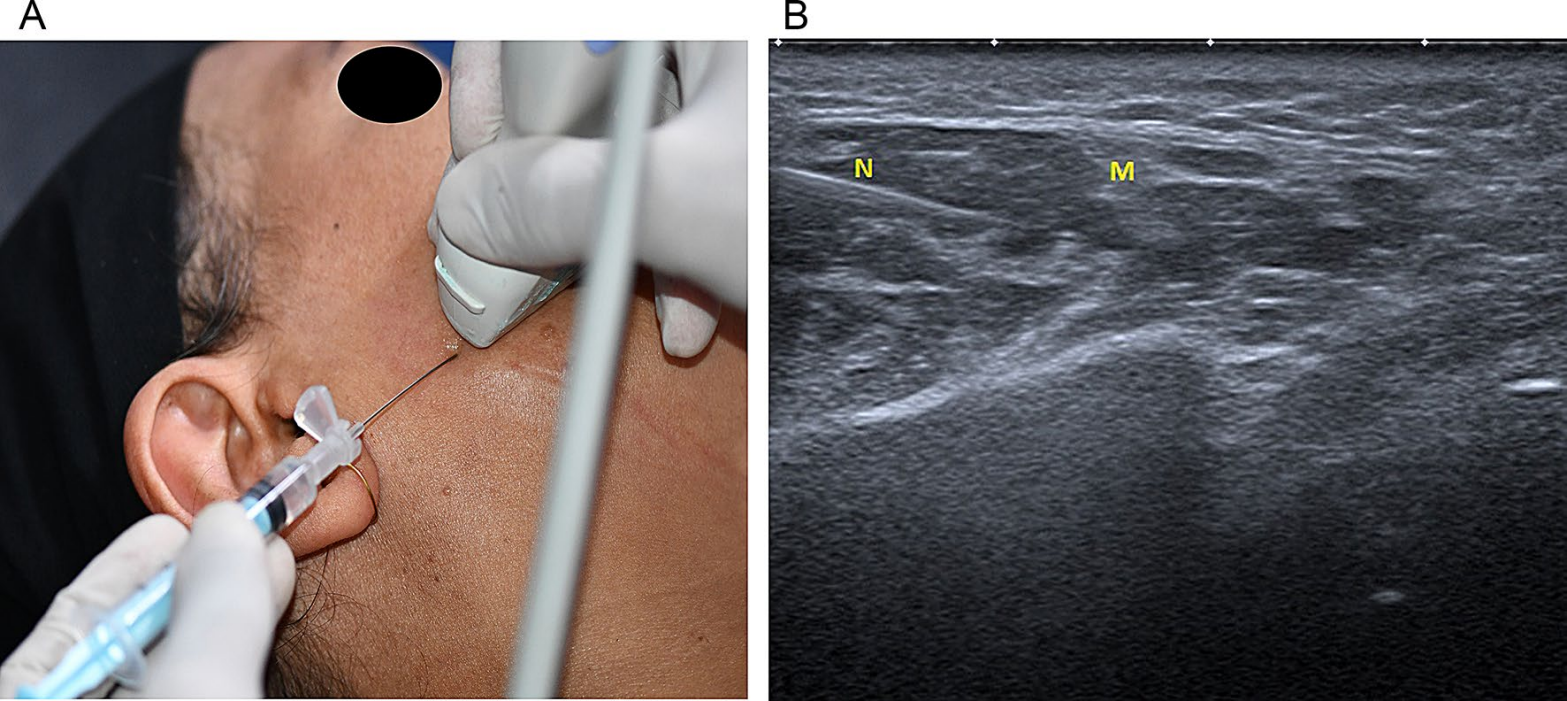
a, b: Showing the extra-oral injection with guided ultrasound (N: needle; M: masseter muscle)
